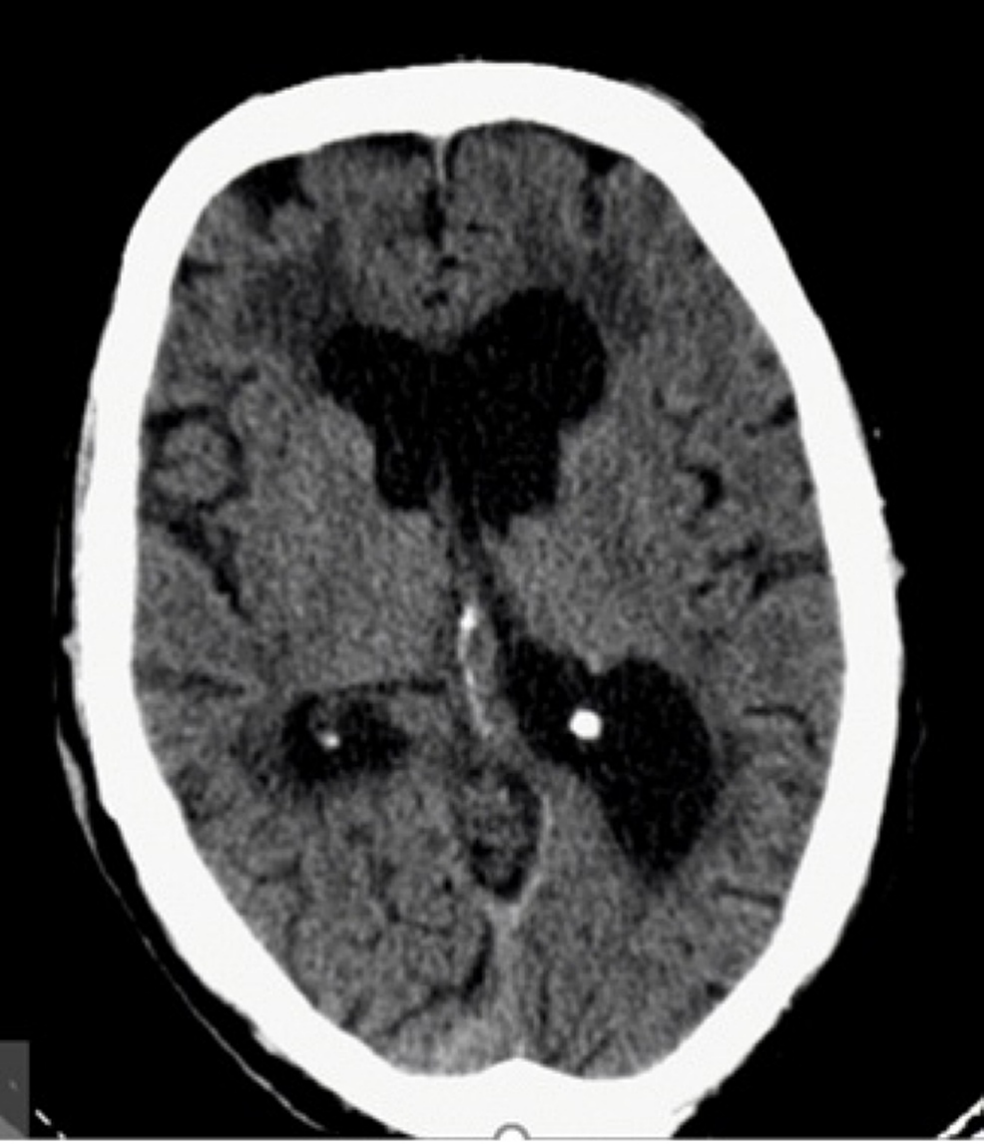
Cranioencephalic CT revealing a cerebral atrophy with subcortical predominance
